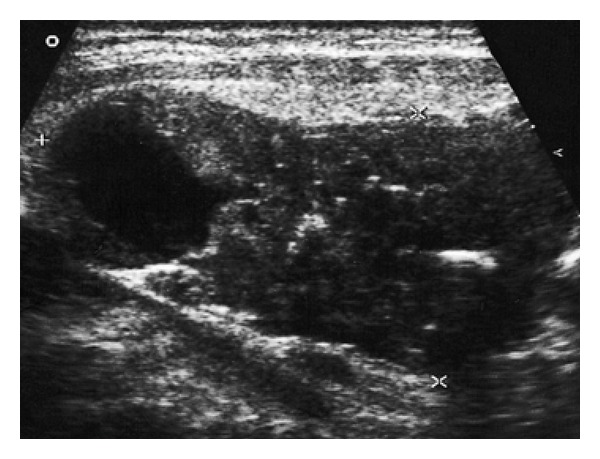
Ultrasound finding of parathyroid carcinoma (longitudinal)—a tumor with cystic degeneration and calcification.
